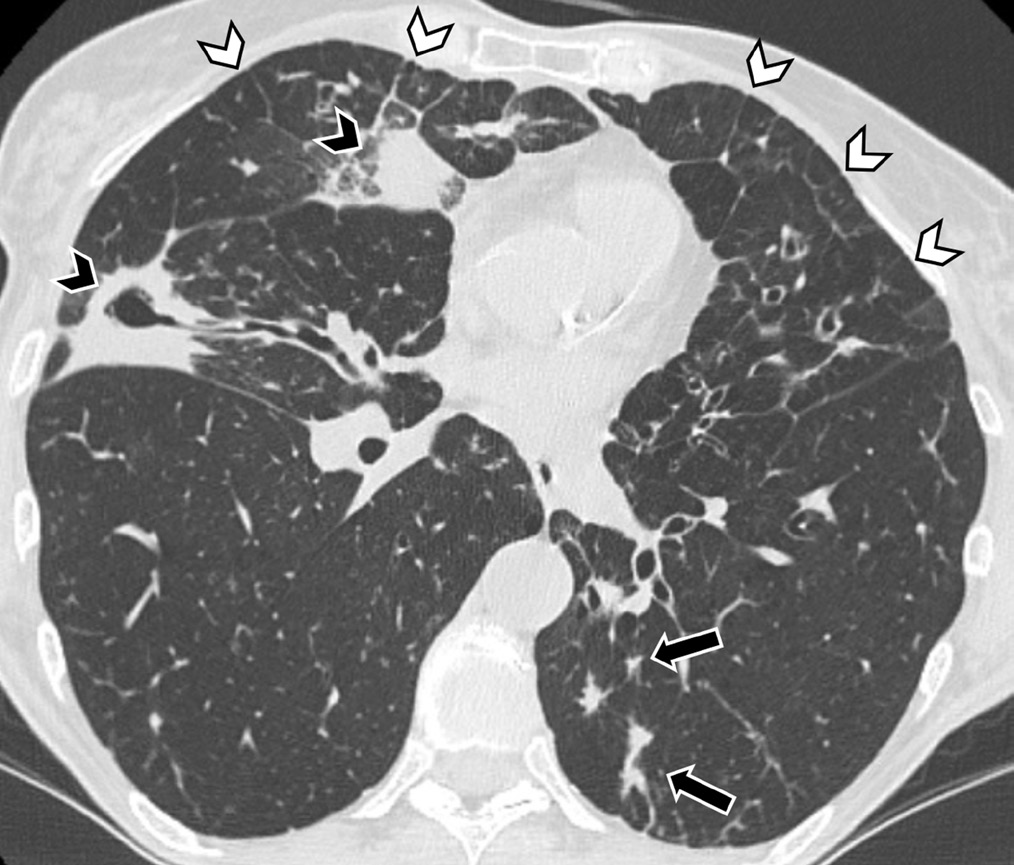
Axial CT image shows extensive bilateral thick-walled bronchiectasis with mucus plugging (black arrows), consolidation (black arrowheads) and thickened interlobular septa (white arrowheads).
